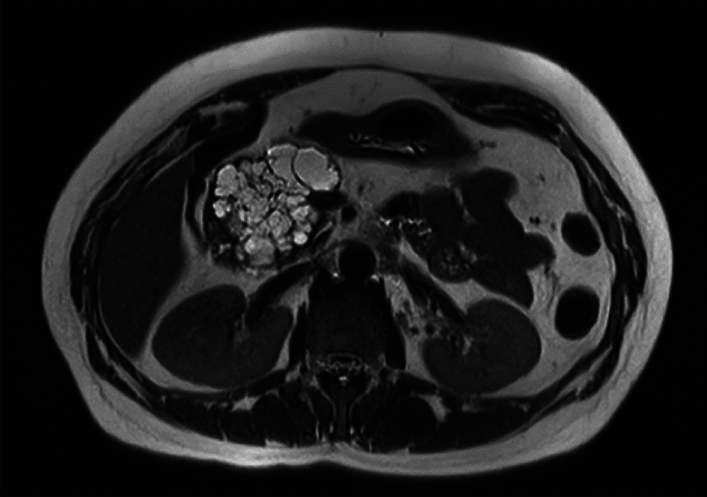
Magnetic resonance imaging (MRI) of the abdomen demonstrating multiple cystic lesions in the pancreas, including a 7.3 multilobulated multiseptated cystic mass at the head of the pancreas, suggestive of serous cystic adenoma (SCA)
